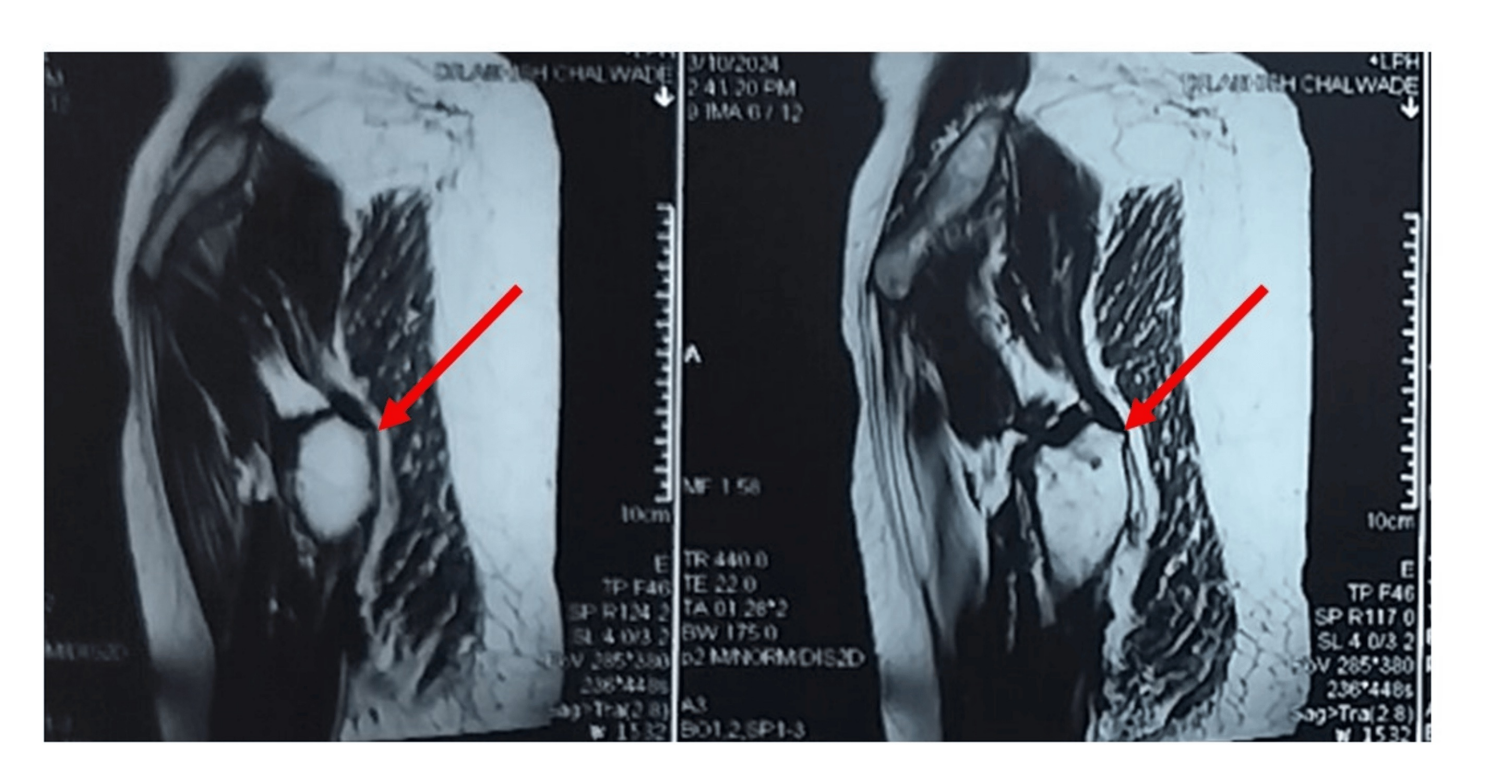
Sagittal MRI pelvis with both hips cuts showing proximal femoral metastasis The red arrow marks shows a lesion in the intertrochanteric area contained in the bony cavity but significantly occupying the proximal femur.
